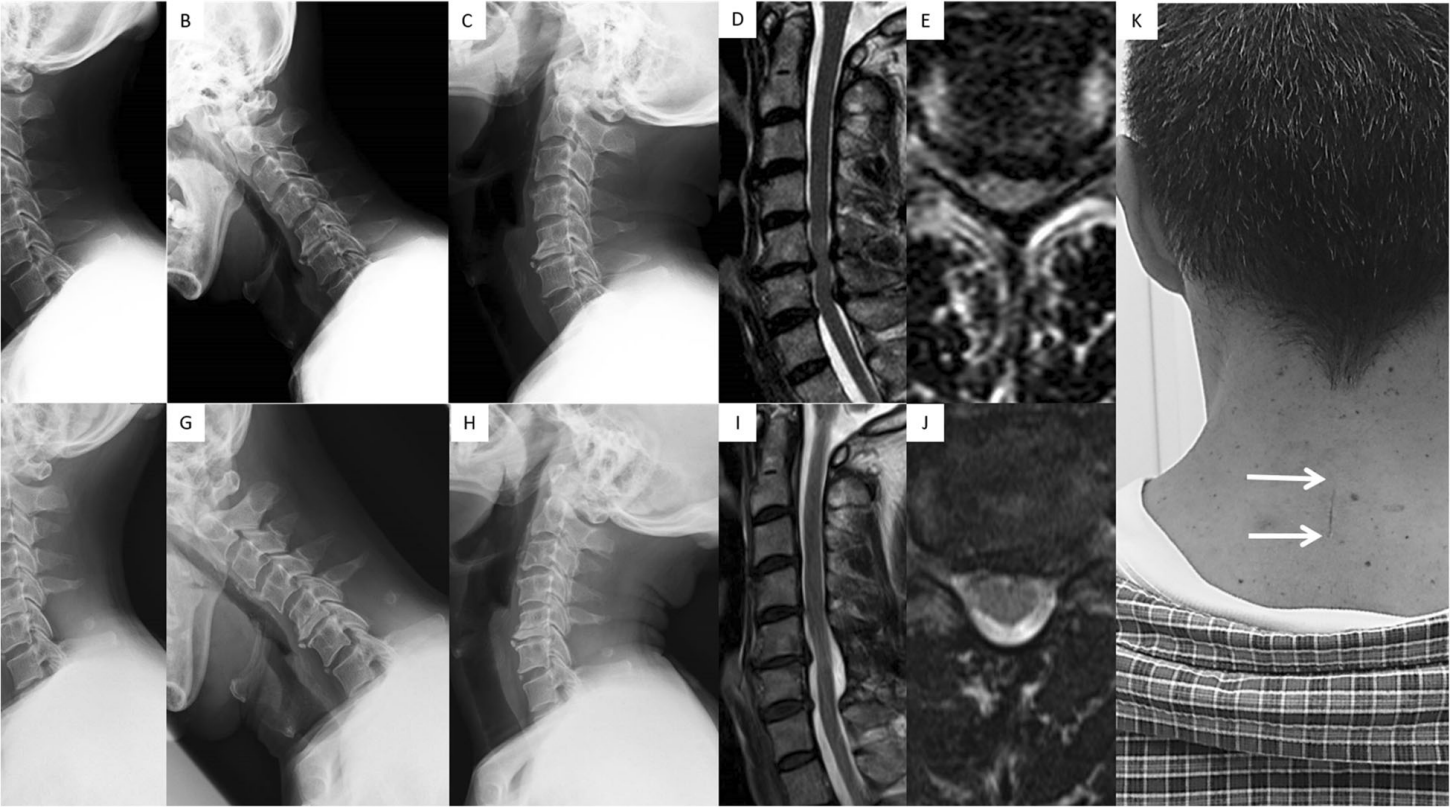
A 58-year-old man presented with two-level spinal cord compression at C5/6 and C6/7 and underwent cervical microendoscopic interlaminar decompression (CMID) from C5 to C7; i.e., laminectomy of C6 and partial laminectomy of C5 and C7 after splitting the C6 spinous process. a, b, c Preoperative lateral X-ray in neutral (a), flexion (b), and extension (c) positions. d, e Preoperative sagittal (d) and axial (e) images on T2-weighted magnetic resonance imaging (MRI). f, g, h Postoperative (at 28 months) lateral X-ray in neutral (f), flexion (g), and extension (h) positions. i, j Postoperative sagittal (i) and axial (j) images on T2-weighted MRI. k Clinical photograph showing the operative scar (arrows). Pre−/postoperative C2/7 Cobb angles, range of motion, spinal cord diameter, and degree of posterior spinal cord shifting were 10/10 degrees, 50/48 degrees, 3.0/5.2 mm, and 2.6 mm, respectively. The pre- and postoperative Japanese Orthopedic Association (JOA) scores were 10 and 16
